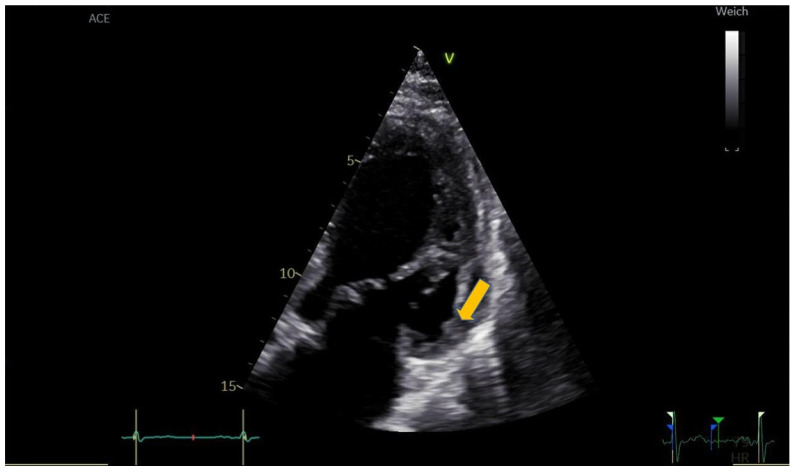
Day 3. Transthoracic echocardiography, modified apical four-chamber view focused on the basal anterolateral wall. A 30 × 8 mm echodense, oscillating structure (arrow) is visible at the basal segment of the anterolateral wall.
